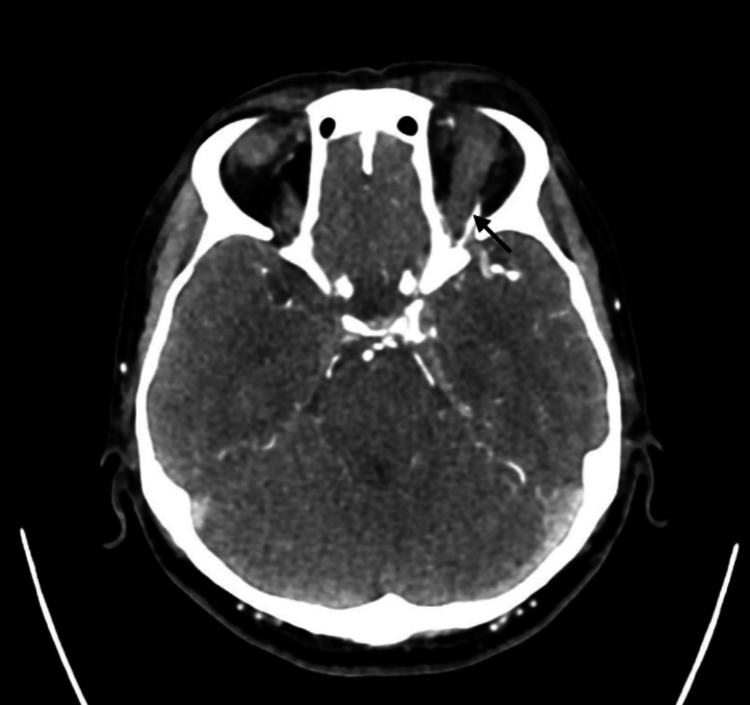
Dilated and tortuous left superior ophthalmic vein (SOV) noted during Computed Tomography Angiography (CTA) of the brain and orbit.
